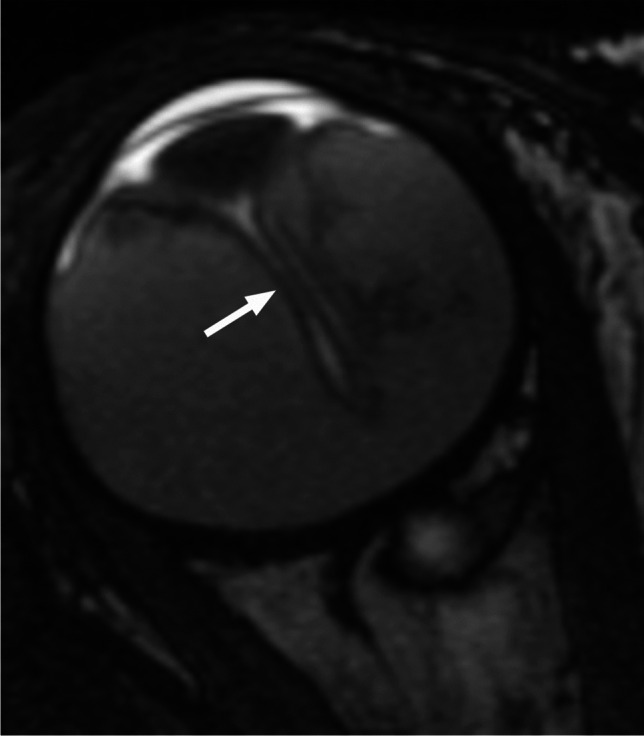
Coats disease (exudative retinitis). High resolution axial T2-weighted image of the globe reveals Y-shaped retinal detachment (arrow), without evidence of underlying mass lesion
